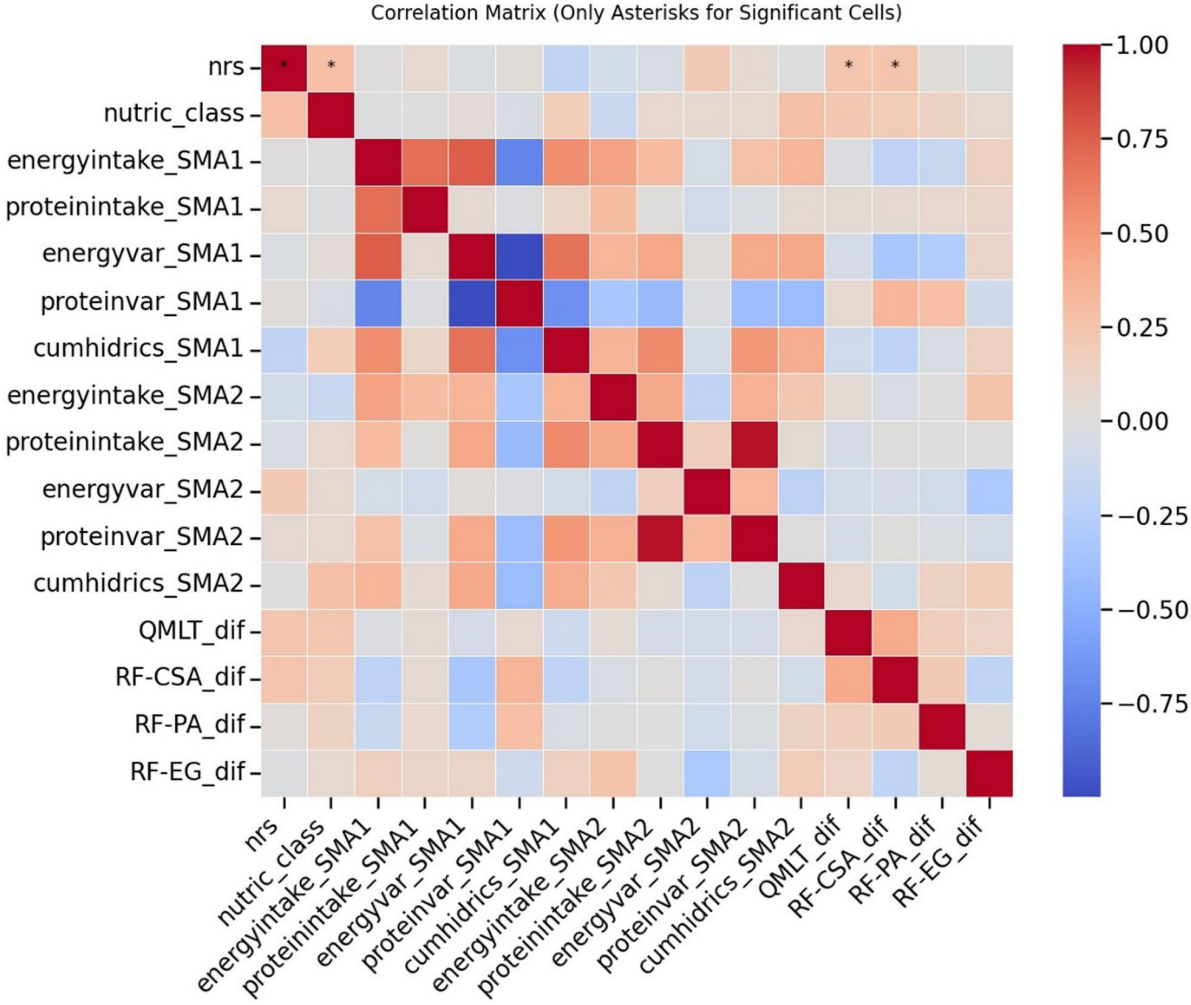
Correlation matrix. Correlation matrix (Pearson’s R coefficients) between skeletal muscle (SM) changes and nutritional support variables. Differences in SM ultrasound measures (e.g., QMLT_dif, RF-CSA_dif) reflect the change from SMA1 to SMA2. Asterisks (*) indicate statistically significant correlations at a threshold of *p* < 0.05. The matrix highlights a significant negative association between NRS 2002 and changes in QMLT and RF-CSA, while no associations were found between nutritional support and SM changes. *QMLT_dif* muscle layer thickness difference between SMA 2 and SMA 1, *RF-CSA_dif* rectus femoris cross-sectional area difference between SMA 2 and SMA 1;
